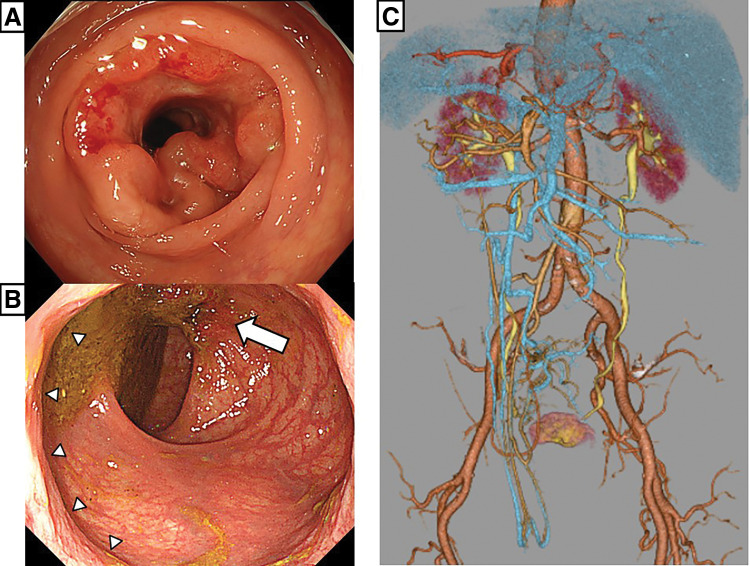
(A) Colonoscopic image: An ulcerofungating-type (Borrmann type II) tumor in the sigmoid colon. (B) An ulcerofungating-type (Borrmann type II) tumor in the lower rectum. (C) Computed tomography angiography showing complete transposition of the abdominal viscera. White arrow: lower rectal tumor; white triangles: upper edge of the anal canal.
